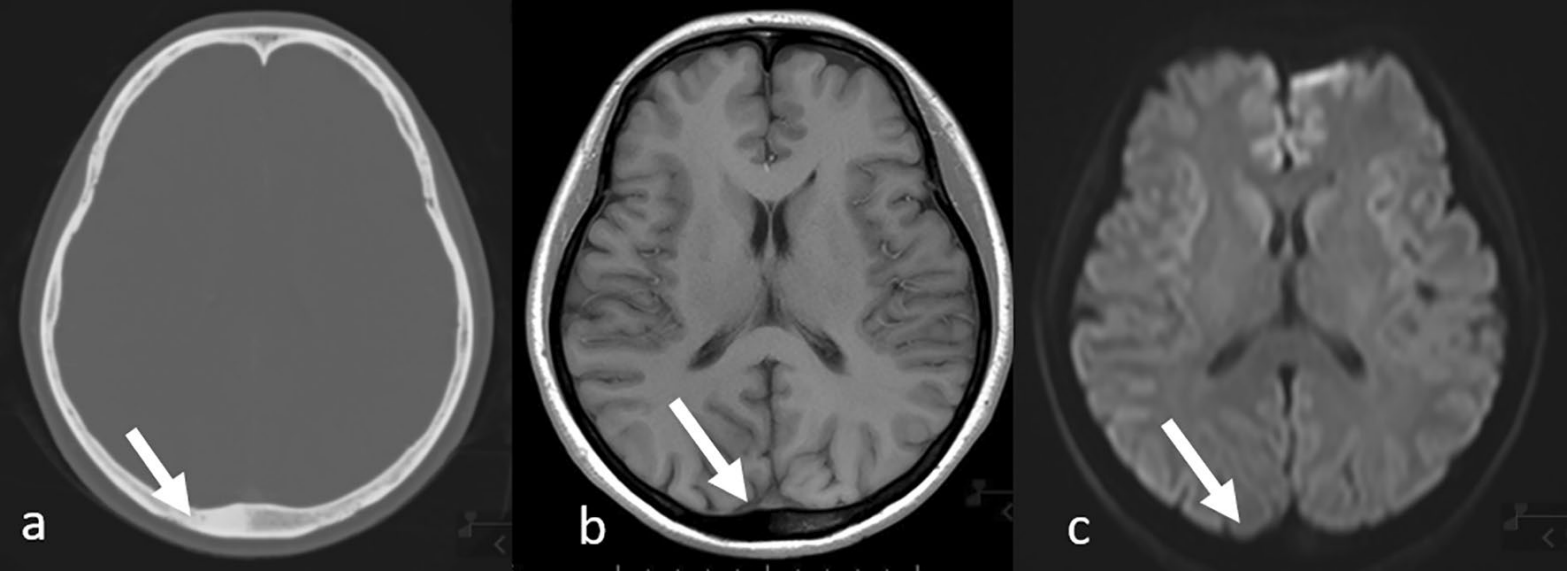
Osteosclerotic skull metastasis in a patient with lung adenocarcinoma. Computed tomography a clearly shows the lesion but it is not clearly visible on MRI: T1-weighted b and diffusion-weighted c images (arrows). *MRI* magnetic resonance imaging
